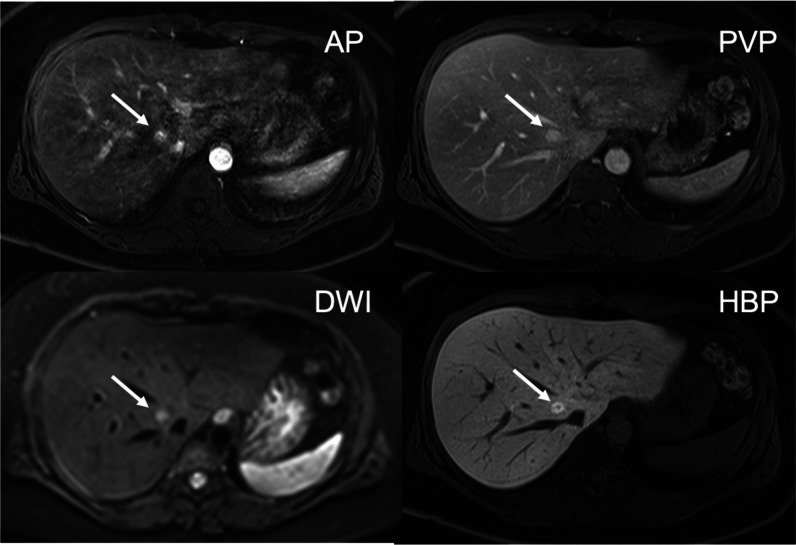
Moderate mixed AP artifacts, score 3, optimal timing, sufficient lesion visibility, score 3: Unsharp appearance of the structures and lesion margins and blurring at organ boundaries due to TSM (score 3) and multiple parallel stripes at high-contrast interfaces, especially in left liver lobe due to TA (score 2): This 33-year-old woman with NASH was referred for MRI because of questionable lesions on ultrasound. The AP shows a small lesion with hyperenhancement in liver segment VIII, despite AP artifacts. In the PVP, the lesion shows contrast retention, and on the 20-min HBP, it has more retention and a central scar. It is uniformly bright on DWI. The findings are consistent with a small FNH
